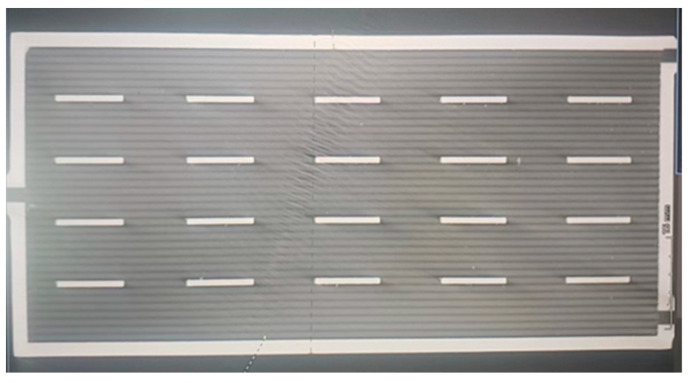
CT scan of the internal support structure of the heat pipe.
